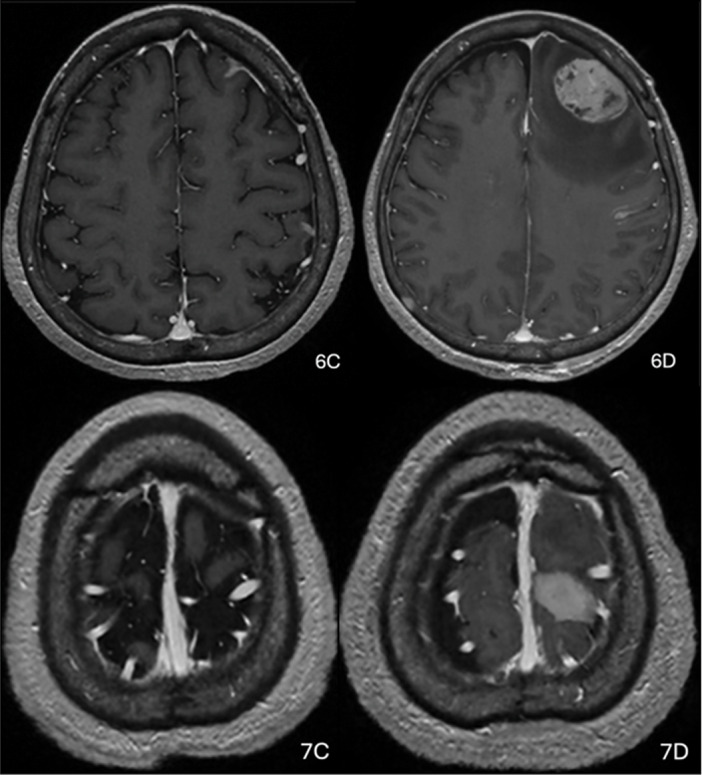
Axial brain MRI scans acquired at four different time points (“A,” “B,” “C,” and “D”). FLAIR sequences are labeled with “1,” while T1-weighted post-contrast images correspond to labels “2,” “4,” “5,” “6,” and “7.” Diffusion-weighted images (DWI), indicating potential diffusion restriction, are labeled with “3.”
